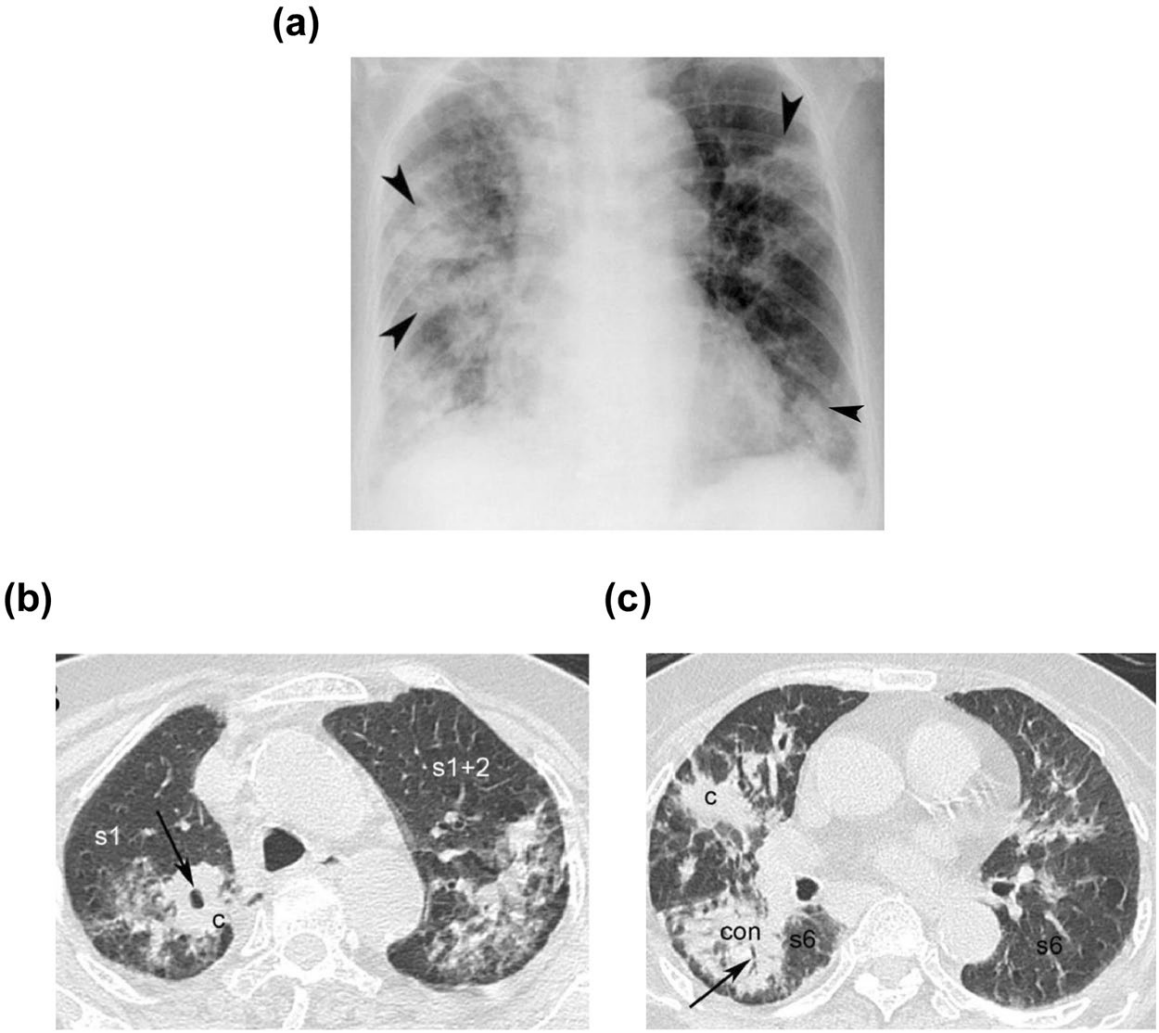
Presentation of an 84-year-male [aPTB] with acute lung injury, presenting as fever (score = 0), dyspnea (score = 0) in HRCT and [CXR + Hypoalbuminemia]; Hypoalbuminemia (score = 1) in [CXR + Hypoalbuminemia] model. The CXR shows consolidation/patch of right/left upper lung field (right/left upper black arrowhead) (score = 1), consolidation/patch of right/left lower lung field (right/left lower black arrowhead) (score = 0) (a). The transverse section of HRCT shows cluster nodules/mass (black arrow) (score = 2) with cavitation (score = 0) in the s1 of right upper lobe, (b) consolidation (black arrow) in the s6 of the right lower lobe (score = 1). (c) The total score in the [CXR (score1) + Hypoalbuminemia (score1)] model is 2, the total score in the HRCT model is 3 [cluster nodules/mass (score = 2) with cavitation (score = 0) in the s1 + consolidation in the s6 of the right lower lobe (score = 1)]. (c) cluster nodules/mass. con:consolidation; s1 = apical segment; s2 = posterior segment right upper lobe; s1 + s2 = apico-posterior segment left upper lobe; s6 = superior segment of right or left lower lobe.
